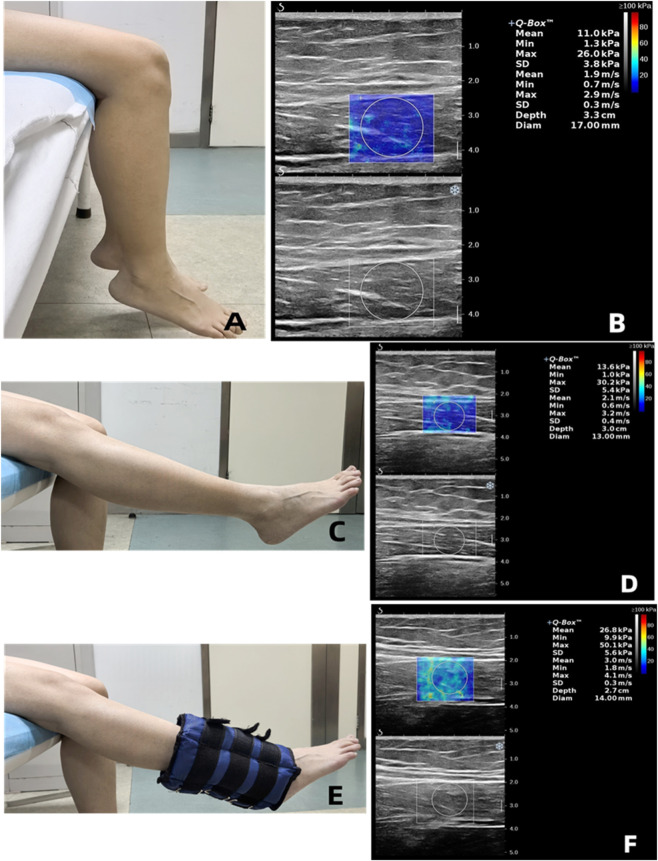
Ultrasound Shear Wave Velocity (SWV) Measurements of the Rectus Femoris in Sitting Position under Different Contraction States. Subjects were in a sitting position. The mean SWV increased from 1.9 to 2.1 m/s to 3.0 m/s in the relaxation (A,B), leg elevated (C,D), and 5-kg loading position (E,F).
